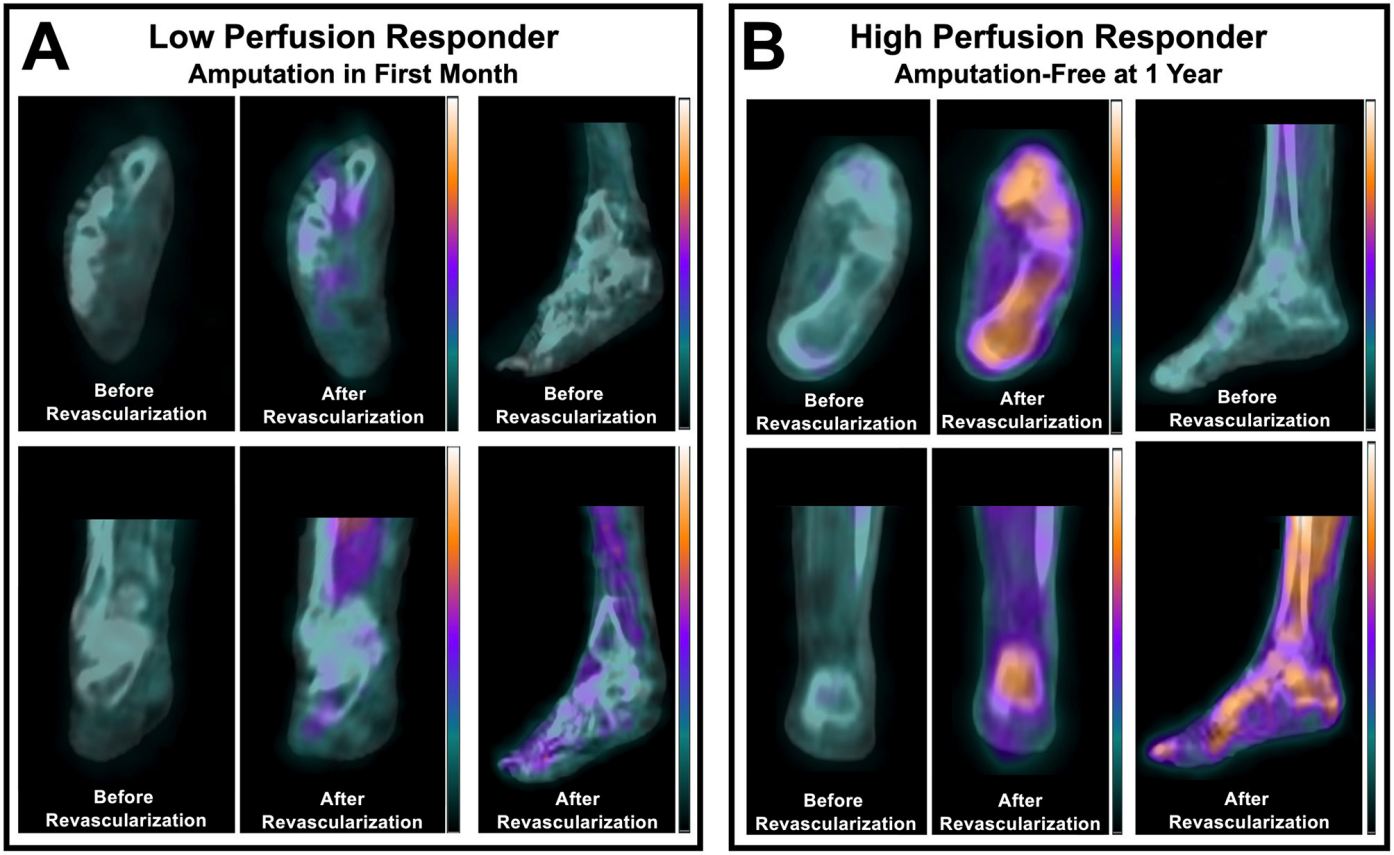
SPECT/CT imaging of the perfusion response to lower extremity revascularization in patients with CLTI. Non-invasive imaging identified differential perfusion responses to peripheral balloon angioplasty in (A) a patient with a low perfusion response who underwent amputation within one month, and (B) a patient who had a high relative perfusion response and remained amputation-free for one year after intervention. Adapted from Chou et al. (22). For a complete description of perfusion imaging methodology, please refer to Chou et al. (22).
